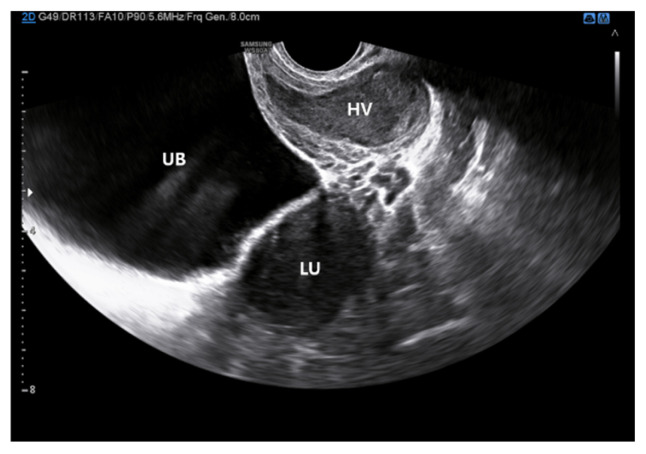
Preoperative transrectal ultrasound image demonstrates the uterus with the obstructed hemivagina of heterogeneous contents. (UB; urinary bladder, LU; uterus, HV; hemivagina).
